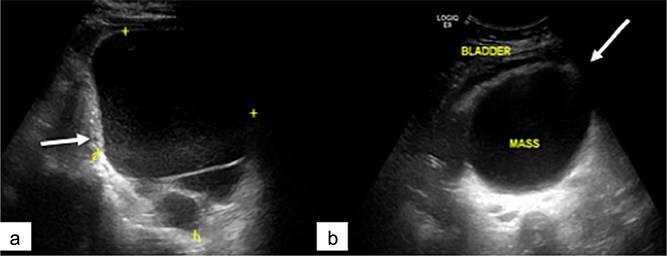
Pelvic ultrasound showing a large multilocular pelvic mass (arrow), displacing the bladder anteriorly.
